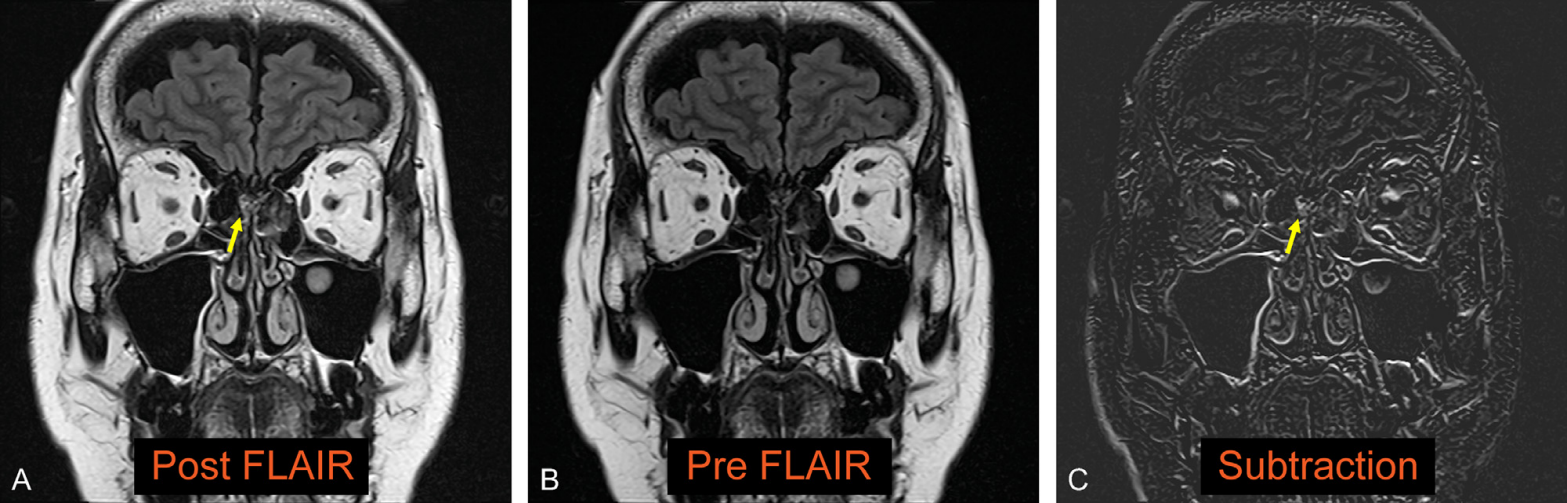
Pre- and postcontrast FLAIR subtraction images show a contrast effect consistent with a protrusion of the right cribriform plate of the ethmoid bone (A-C, arrow).
